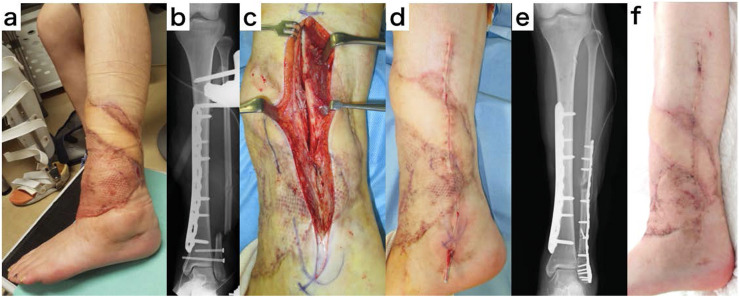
Treatment course of case 2. a. Appearance before secondary surgery. b. X-ray before secondary surgery. c. Appearance after provisional incision. d. Appearance after rough suture. e. X-ray after secondary surgery. f. Appearance after wound healing.
